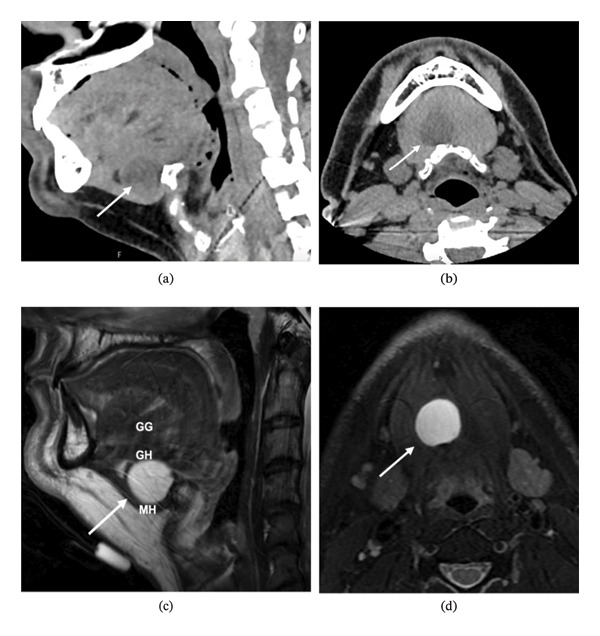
(a) Sagittal and (b) axial contrast‐enhanced computed tomography demonstrating a cystic lesion (arrows) in the sublingual space, deep to the mylohyoid muscle. (c) Sagittal and (d) axial T2 weighted magnetic resonance imaging of the same lesion (arrows); genioglossus (GG), geniohyoid (GH), and mylohyoid (MH).
